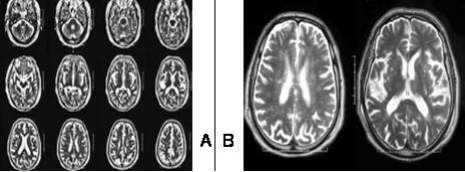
Two independent MRI showed leukoaraiosis in T2 weighted images while diffusion images were normal. Never acute ischemic lesion was observed. a) MRI performed in the forth day of evolution. b) Detail of the MRI performed in the 76^th ^day.
